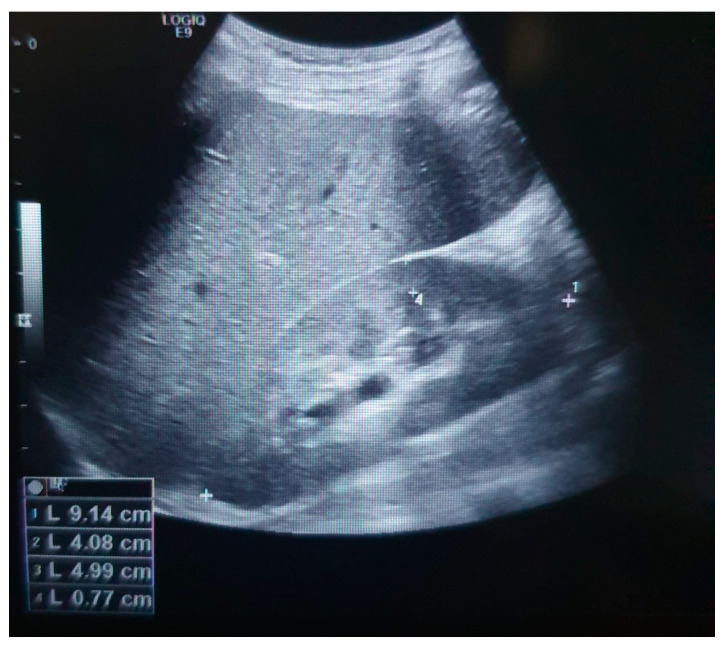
Renal ultrasound showing mild pyelocalyceal dilation.
